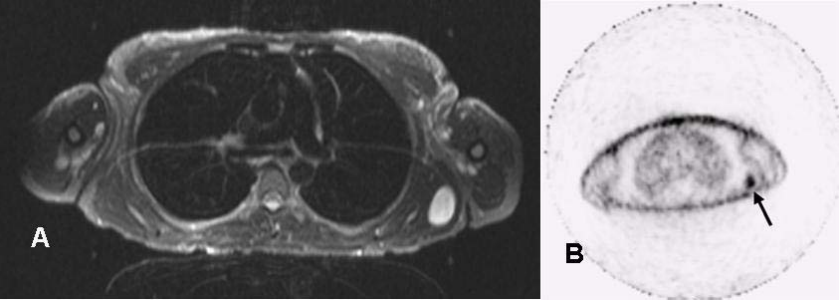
Magnetic resonance imaging A) Transaxial MRI scan (STIR, 5 mm slice thickness, left) B) Corresponding ^18^FDG PET scan (5 mm slice thickness, right). Increased FDG uptake is seen in a subcutaneous lesion in the left shoulder region (arrow).
